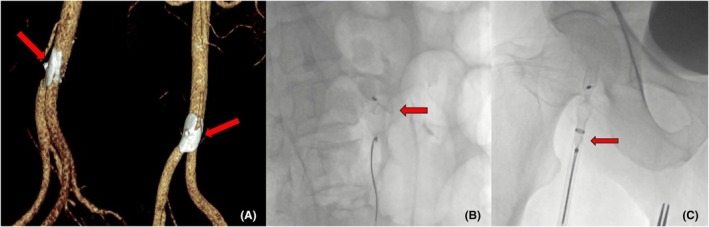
Detection of the Embolized Device via CT and retrieval using a snare catheter. (A) The device is observed in the terminal aorta. (B) The snare catheter is seen capturing the proximal portion of the device. (C) The device, secured by the snare, is being retracted into the 14F sheath.
